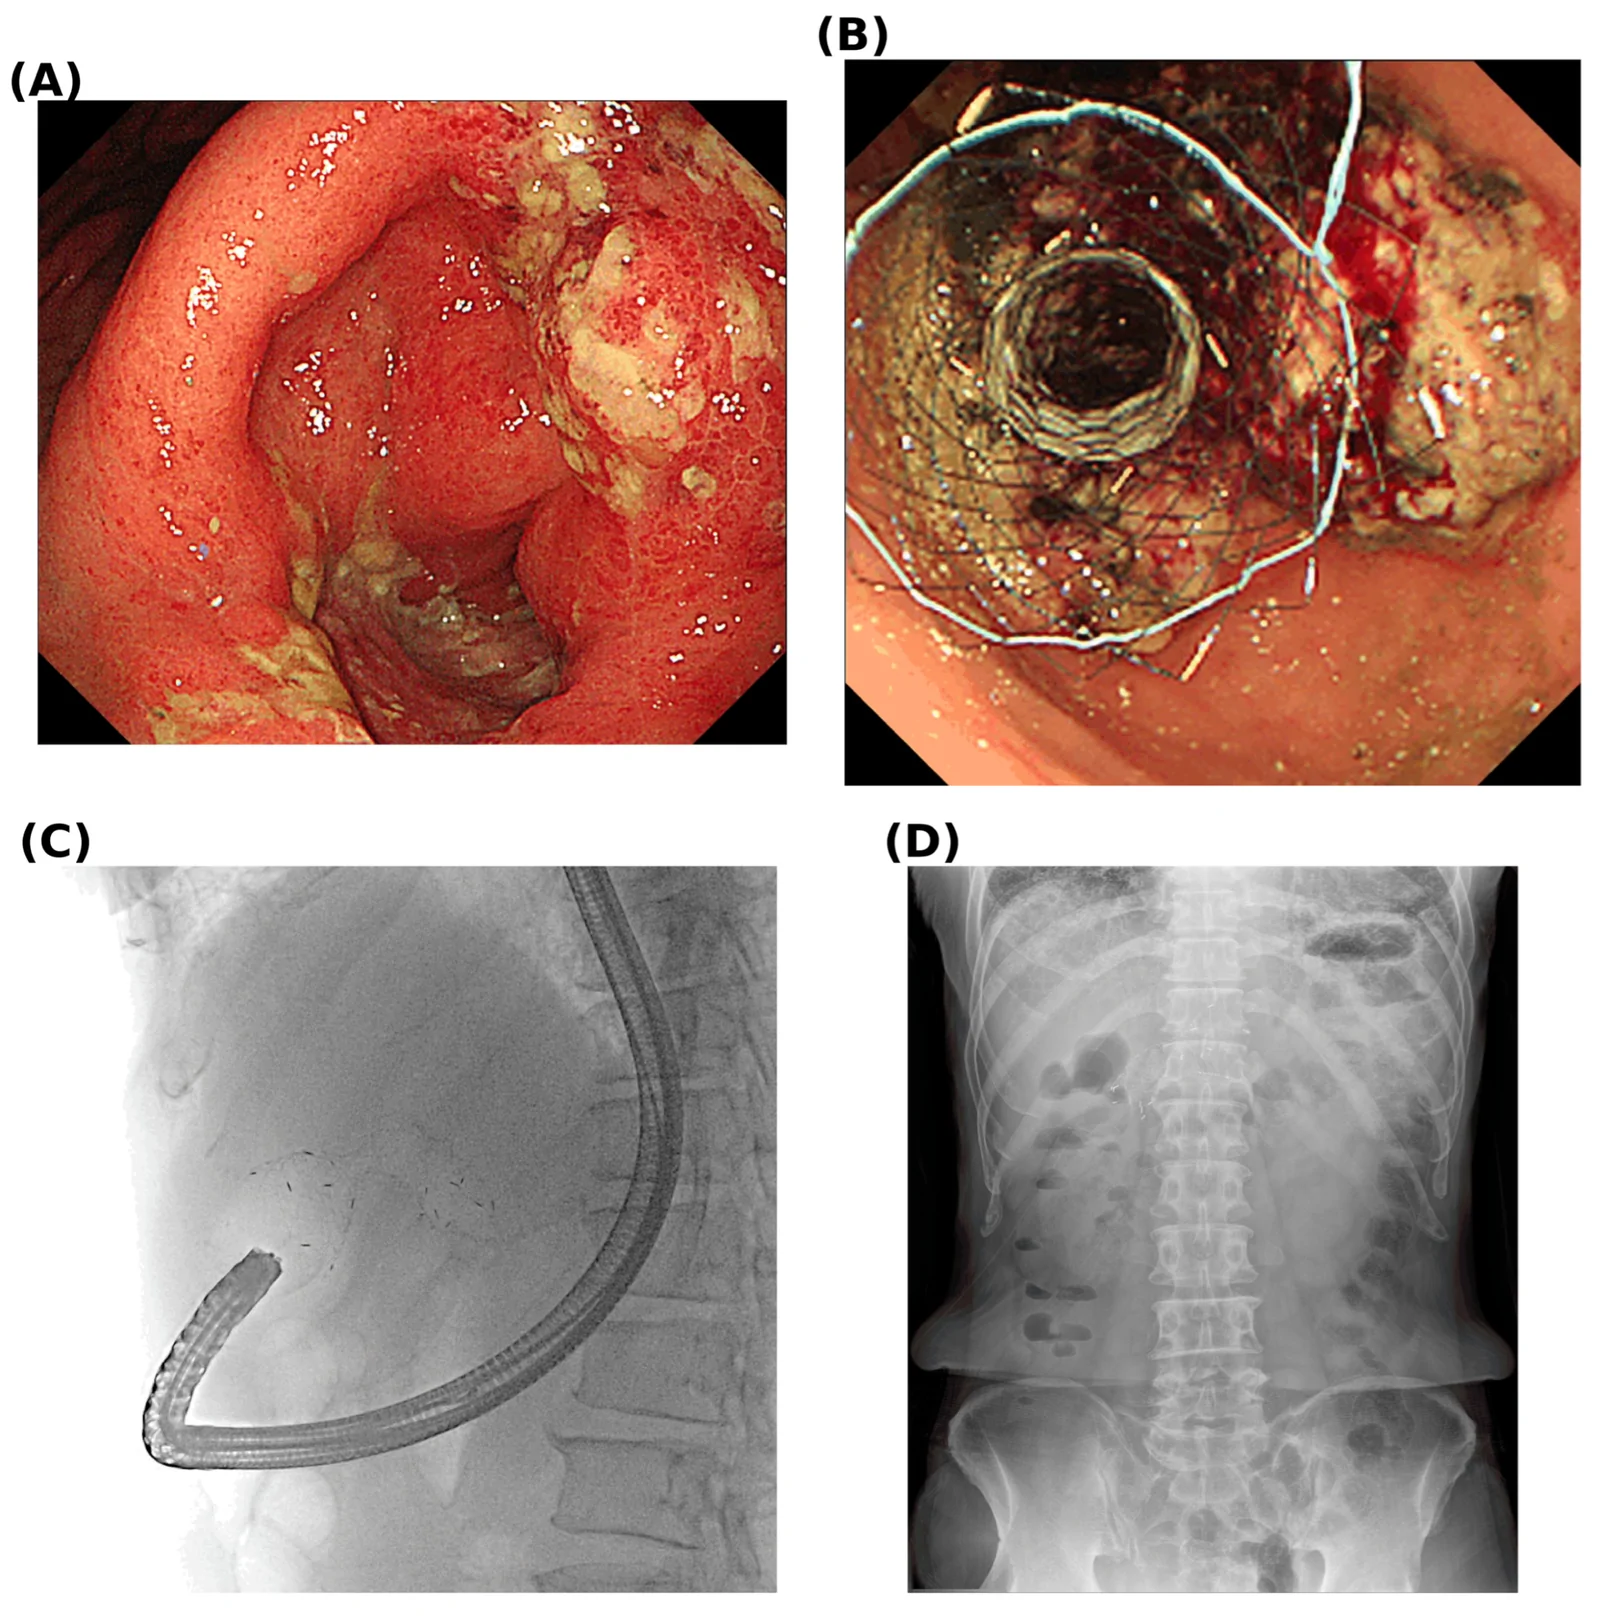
Endoscopic and fluoroscopic images of partially covered duodenal stent placement. Representative images of partially covered duodenal stent (HANAROSTENT Duodenum/Pylorus Kim’s Flare [NCN]; M.I.Tech) placement for malignant gastric outlet obstruction caused by gastric adenocarcinoma. (A) Severe luminal stenosis of the gastric outlet before stenting. (B) Proximal flare of the partially covered stent spanning the gastric outlet immediately after deployment. (C) Fluoroscopic image during deployment showing the radiopaque markers and stent outline across the malignant stenosis. (D) Abdominal radiograph obtained within 48 h after stenting, confirming stent expansion and restoration of luminal patency.
